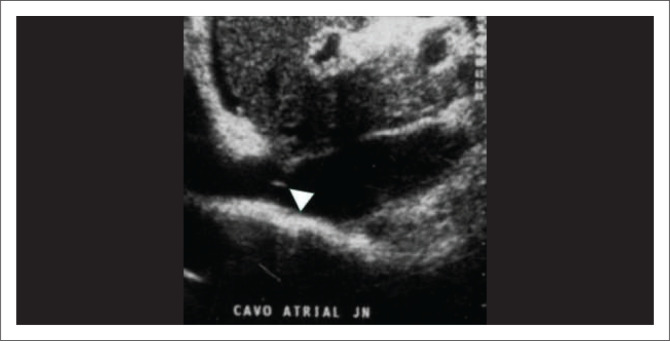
A 34-year-old male who was evaluated for Budd-Chiari syndrome was found to have a web (arrowhead) in the inferior vena cava at the level of cavoatrial junction on ultrasonography.
